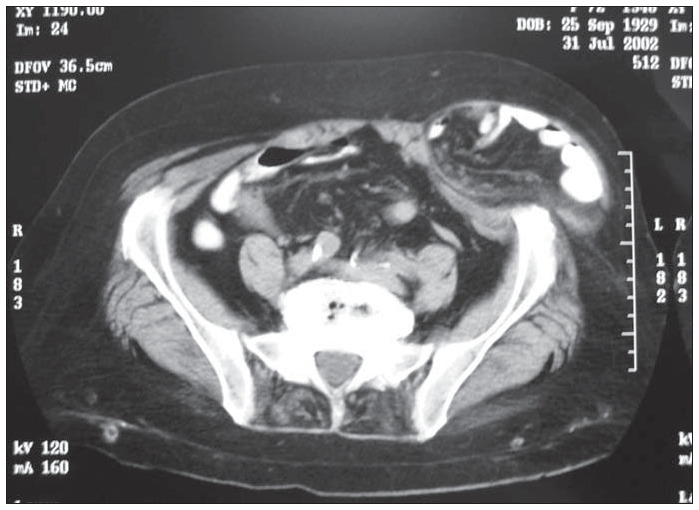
Abdominal computed tomography scan in a 72-year-old woman with a Spiegel incarcerated hernia: fluid and air-filled mass in the soft tissue area of the lower left-side abdominal wall.
